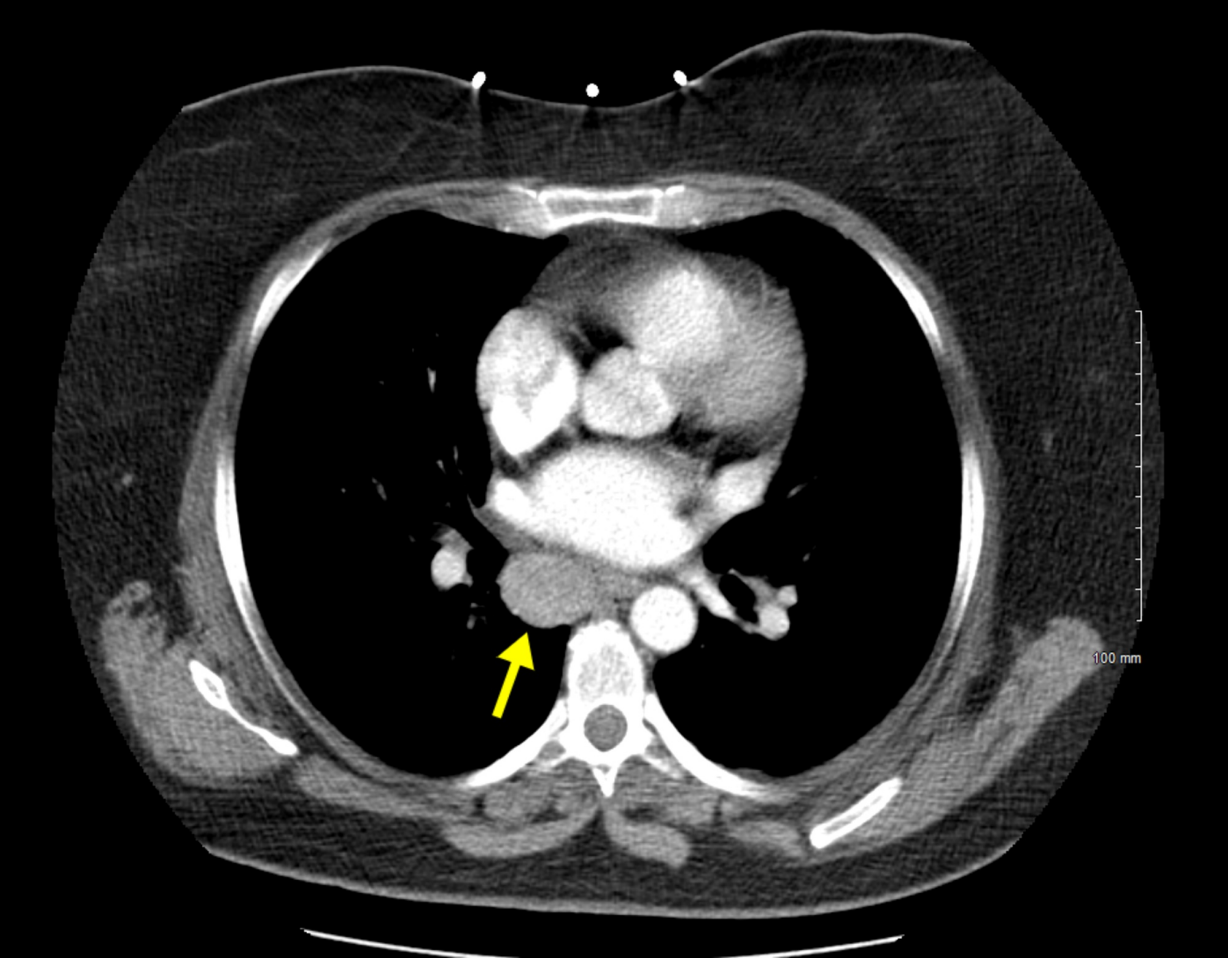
Computed tomography (CT) of the abdomen and pelvis 2.4 cm x 2.0 cm soft tissue mass in the right paraesophageal location abutting the esophagus as well as the left atrium in the lower chest region.
